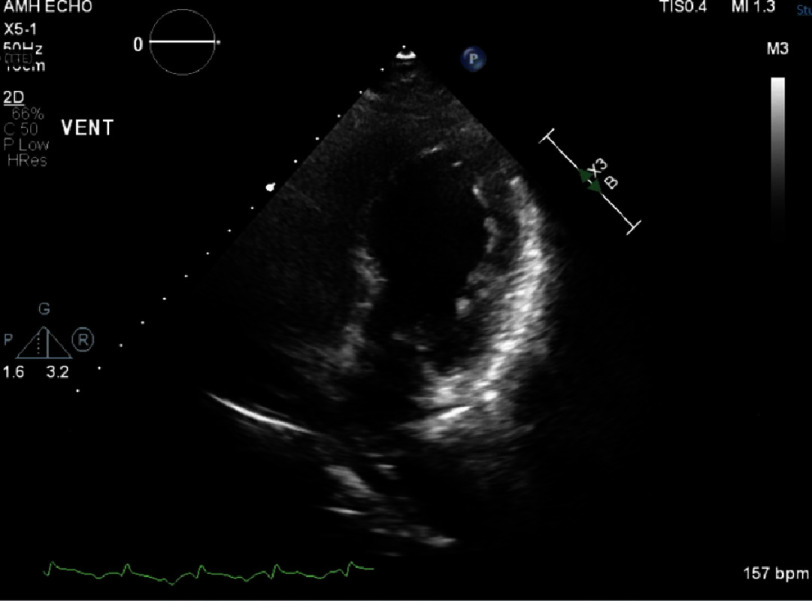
Transthoracic echocardiogram with two chamber views demonstrates apical akinesis and ballooning of the left ventricle.
